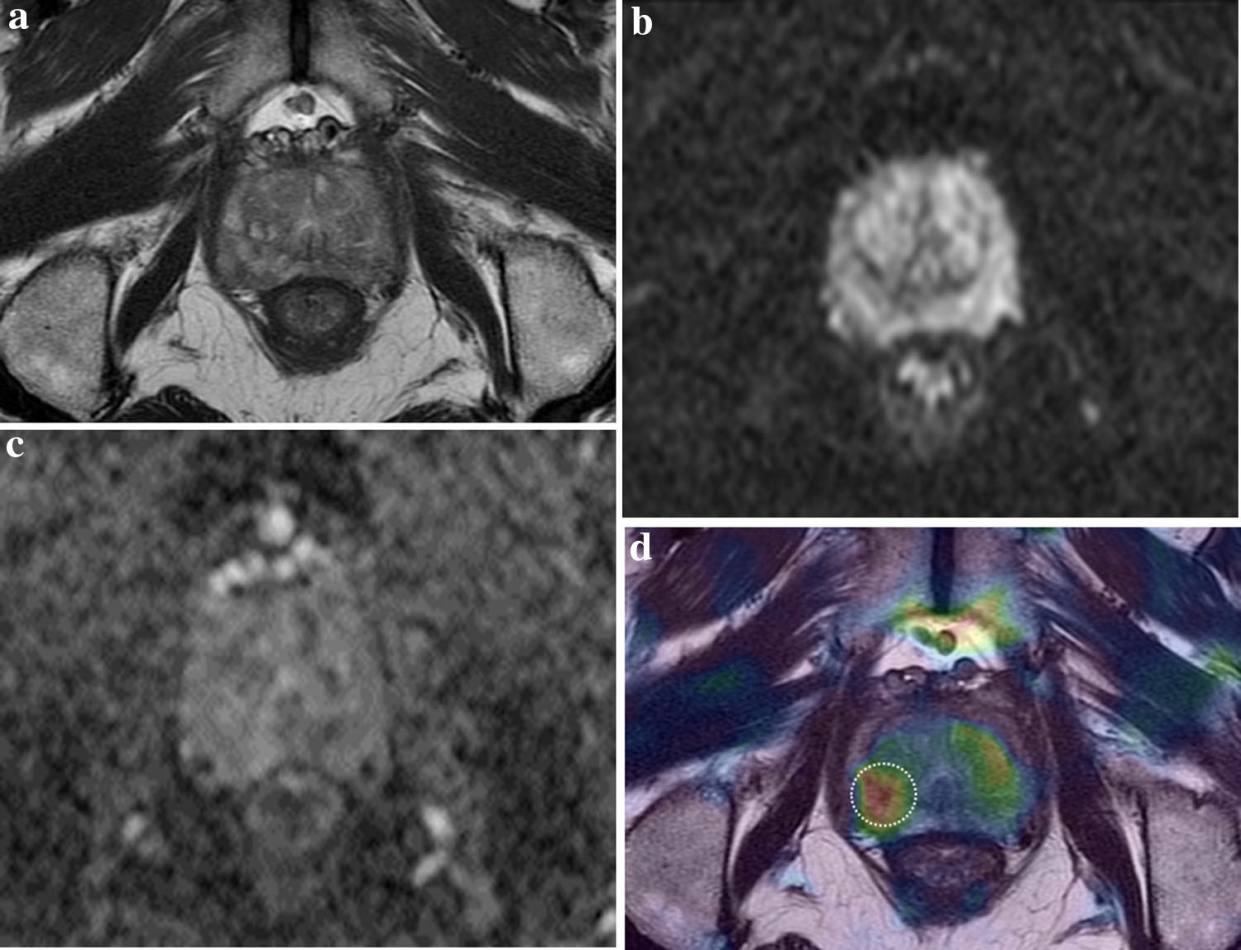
A 64-year-old man was diagnosed with prostate cancer in the right lobe after radical prostatectomy. (a–c) There is no evidence of clinically significant prostate cancer on conventional prostate MRI (from left to right: axial T2WI, DWI, and ADC). (d) Elastogram shows a tumor in the right apical peripheral zone of the prostate, with a mean stiffness of 7.512 kPa.
